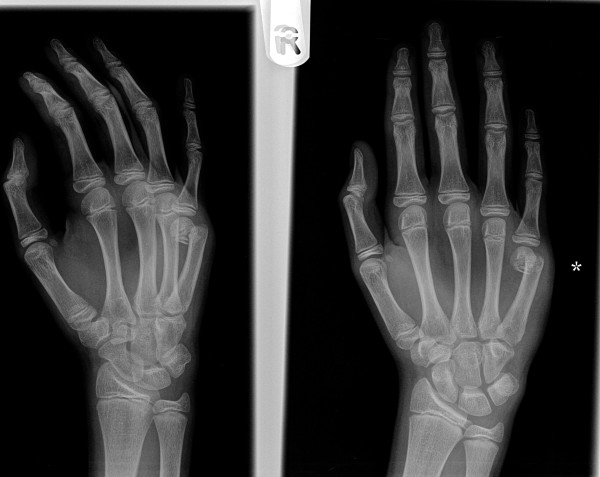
Radiograph of a 16 year old male patient with displaced little finger metacarpal neck fracture.
